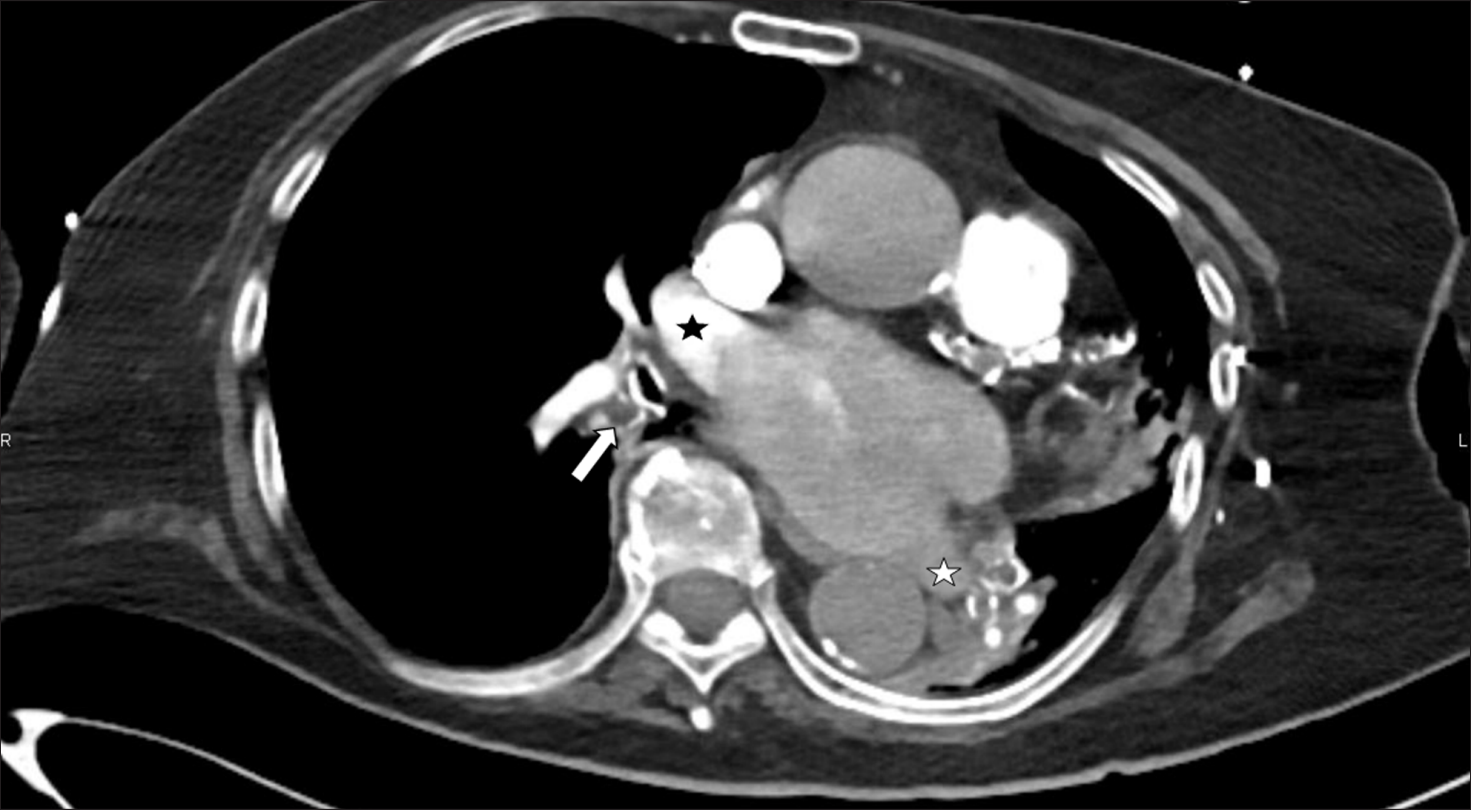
Axial CT of the right lower lobe bronchus that is filled with mucus (arrow), also showing unusual lack of opacification of the left atrium, where only the right superior pulmonary vein is opacified (black star). The left inferior pulmonary vein is not opacified (white star).
